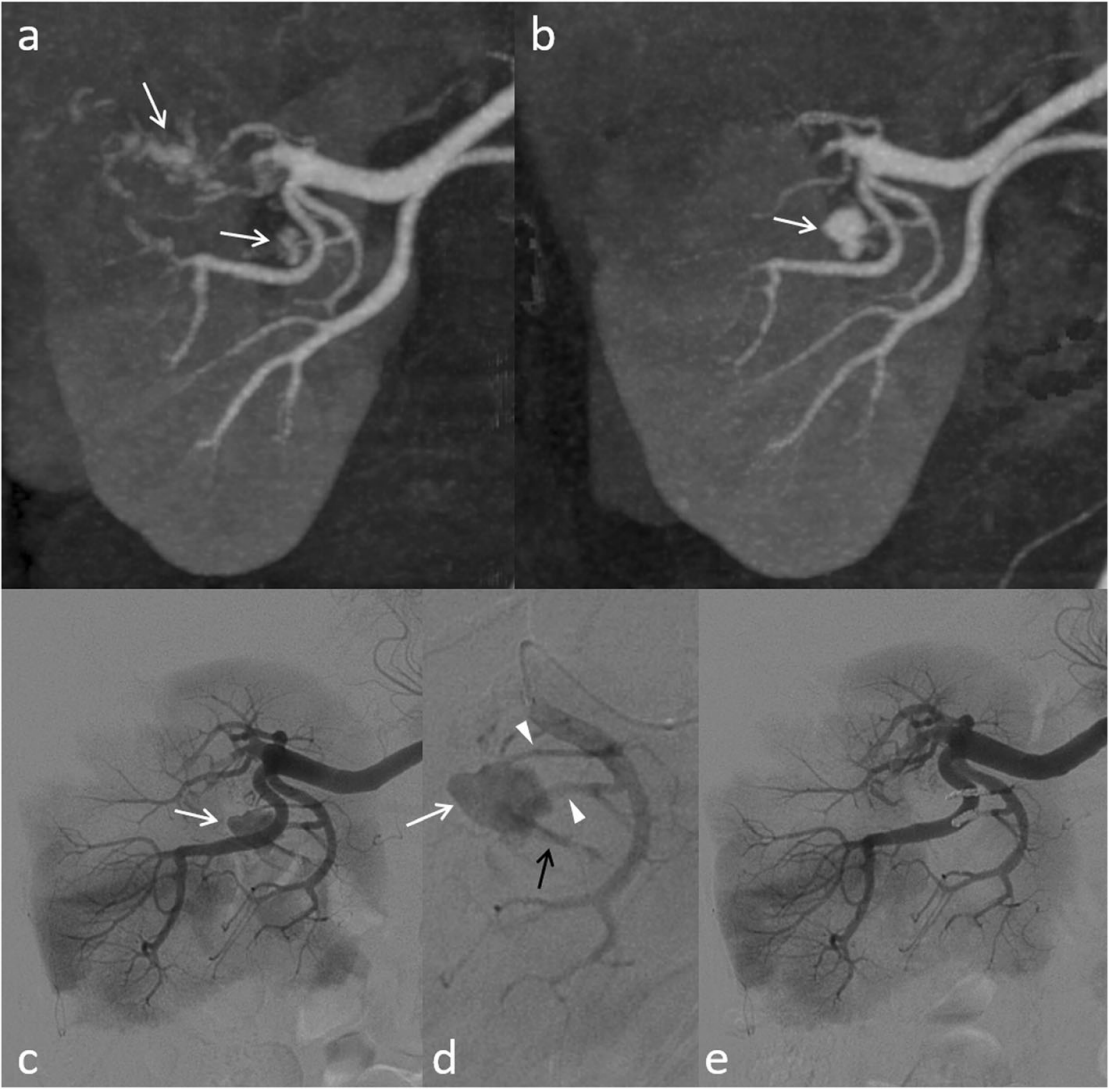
A case of a developing renal artery pseudoaneurysm (RAP), with symptoms, in a 54-year-old woman. a Irregularly shaped enhancements are observed (arrows) on early postoperative contrast-enhanced CT on postoperative day (POD) three. b The larger one disappeared and the smaller one increased in size developing into RAP (arrow) on CT on POD seven, with sudden hematuria. c, d, e Angiography shows two branches (arrowheads) that feed the RAP (white arrows) with extravasation into the renal pelvis (black arrow), which are successfully embolized using microcoils on POD eight
